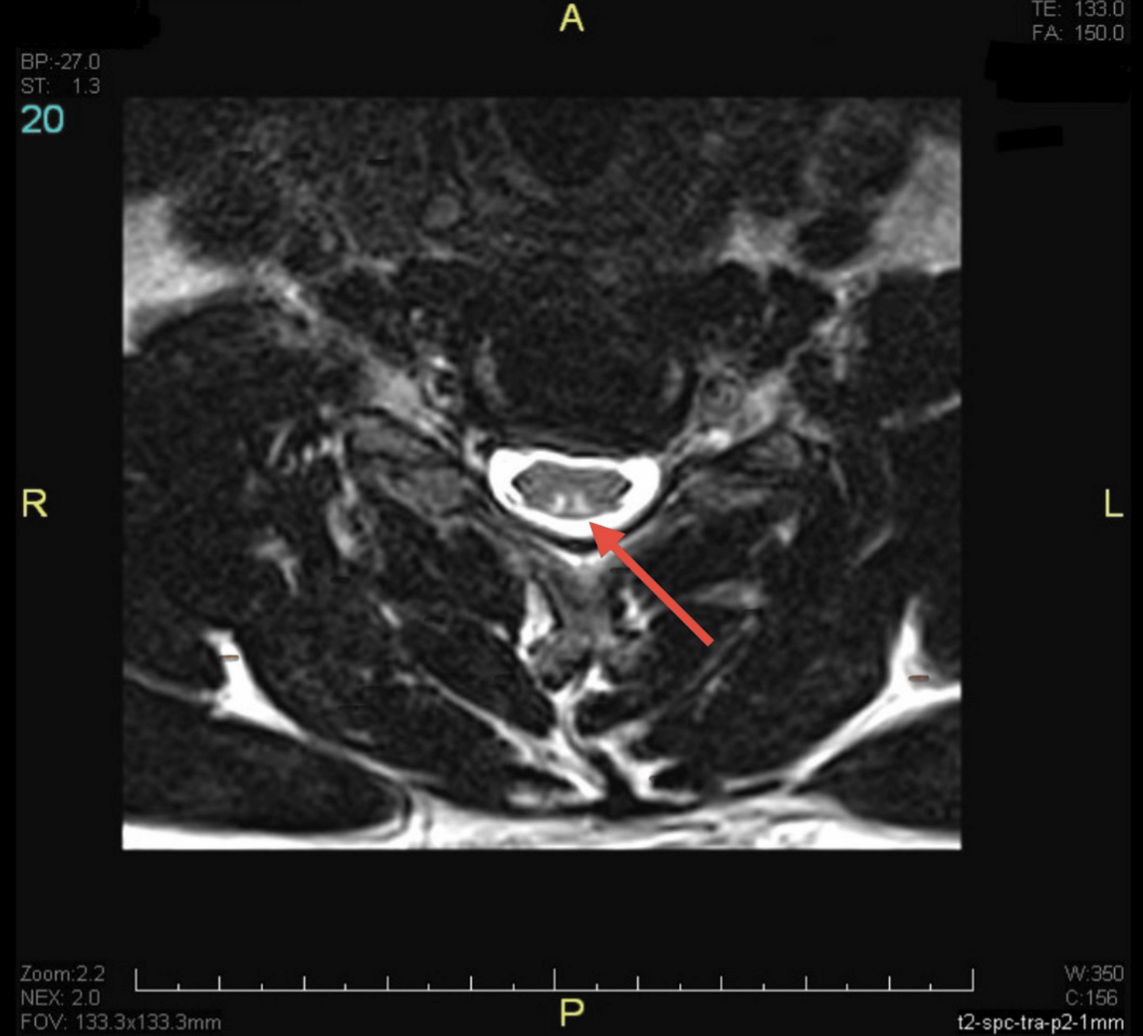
Axial T2-Weighted MRI of Cervical Spine Showing Posterior Column Involvement Axial T2-weighted MRI at the C3 level reveals bilateral symmetrical hyperintensity in the dorsal columns (red arrow), forming the characteristic “inverted V sign” seen in subacute combined degeneration due to vitamin B12 deficiency. The anterior cord is spared.
